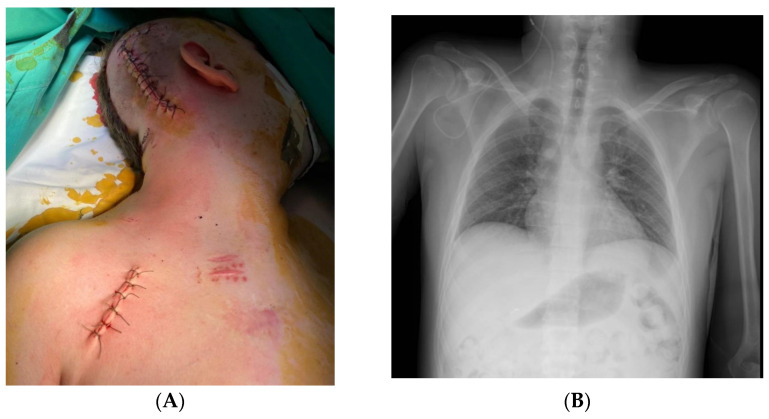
Postoperative clinical aspect (A) and postoperative X-ray aspect of the right ventriculo-subclavian shunt (B).
